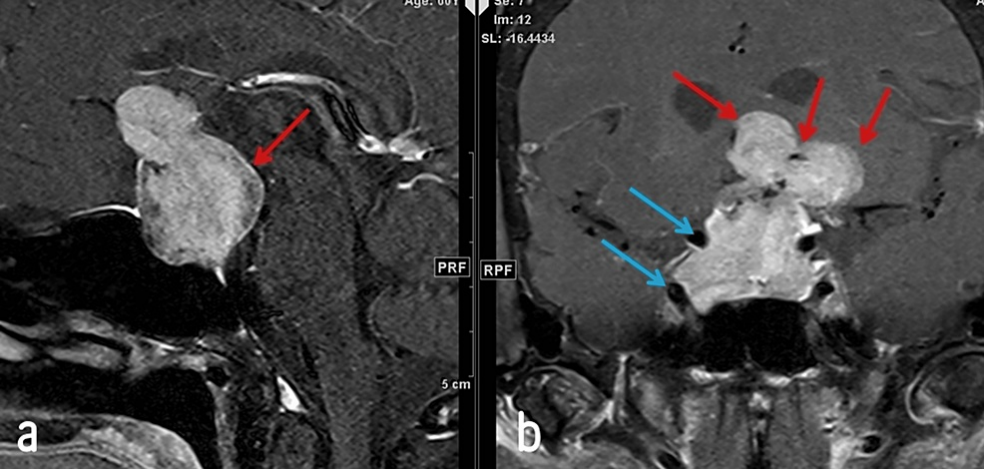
Enhanced T1-weighted fat-saturated brain MRI in both sagittal (a) and coronal (b) views revealing a mass causing significant compression on the hypothalamus and optic chiasm (red arrow). Additionally, an encasement of the internal carotid arteries is observed without any narrowing or occlusion (blue arrows). No cystic component is detected
